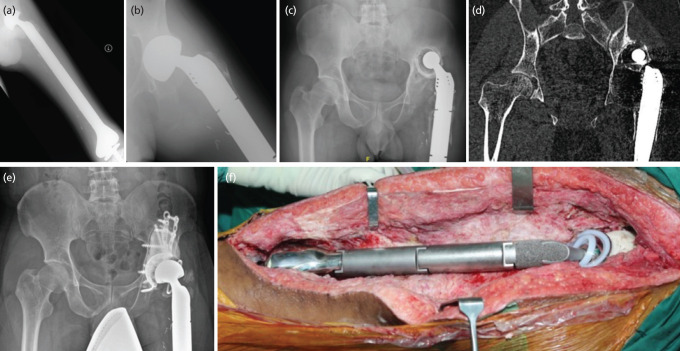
Case 3. A 16-year-old boy, diagnosed with distal femur osteosarcoma and medullary extension to the trochanteric region. Wide resection and total femur endoprosthesis were done at the age of 16-year-old. The bipolar femoral head had to be converted to cemented liner due to early cartilage erosion. He came eight years later with limb shortening and an unstable hip due to superolateral erosion of the acetabulum. The hip was reconstructed with cemented Gap II anti protrusio cage and constraint cup for the stability of the hip. After ten years of revision, he is now ambulating pain-free and has a stable hip. (a, b) Total femur endoprosthesis with bipolar head showed acetabular erosion. (c, d) Radiograph and CT scan showed polyethylene liner had migrated superiorly with significant bone loss, (e) Cemented anti protrusio cage with constraint cup. (f) Intra-operative photo showed cemented constrained cup with bipolar articulation.
